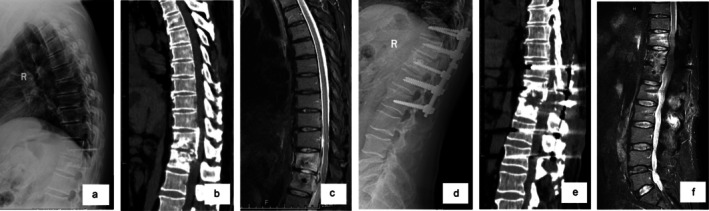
A 65‐year‐old male patient diagnosed with T11‐12 vertebral tuberculosis after T11‐12 vertebral augmentation. (a–c) Preoperative X‐ray, CT, and MRI revealed bone destruction in the T11‐12 vertebrae and intervertebral space; the bone cement filled the vertebral body well, with partial cement leakage into the spinal canal. After 2 weeks of standard antituberculosis drug treatment, posterior thoracic canal decompression, posterolateral bone graft fusion, and internal fixation were performed; (d and f) Postoperative 18‐month follow‐up X‐ray, CT, and MRI demonstrated well‐positioned internal fixation without obvious loosening. No progressive bone destruction was observed in the T11‐12 vertebrae compared with the preoperative period, and the focal lesion achieved favorable healing.
